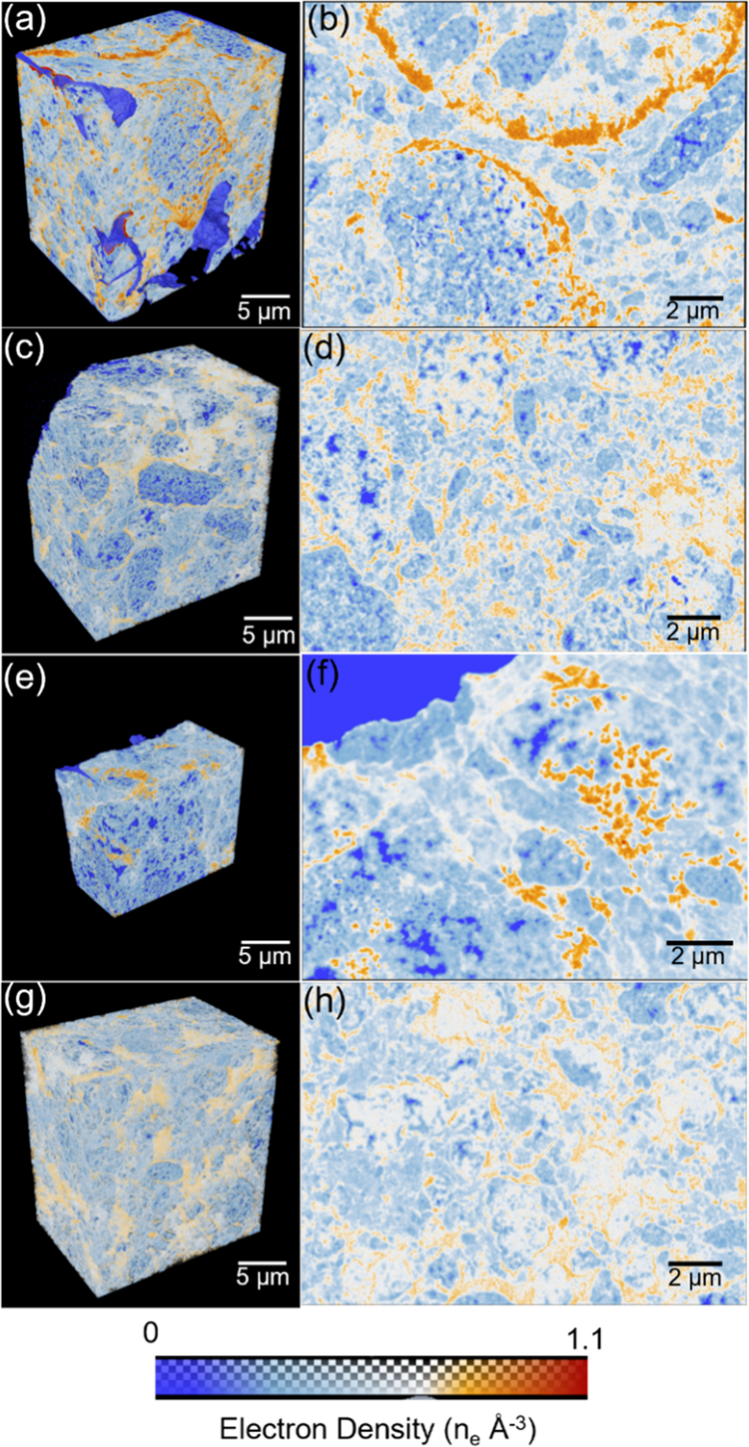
Ptychographic X-ray computed
